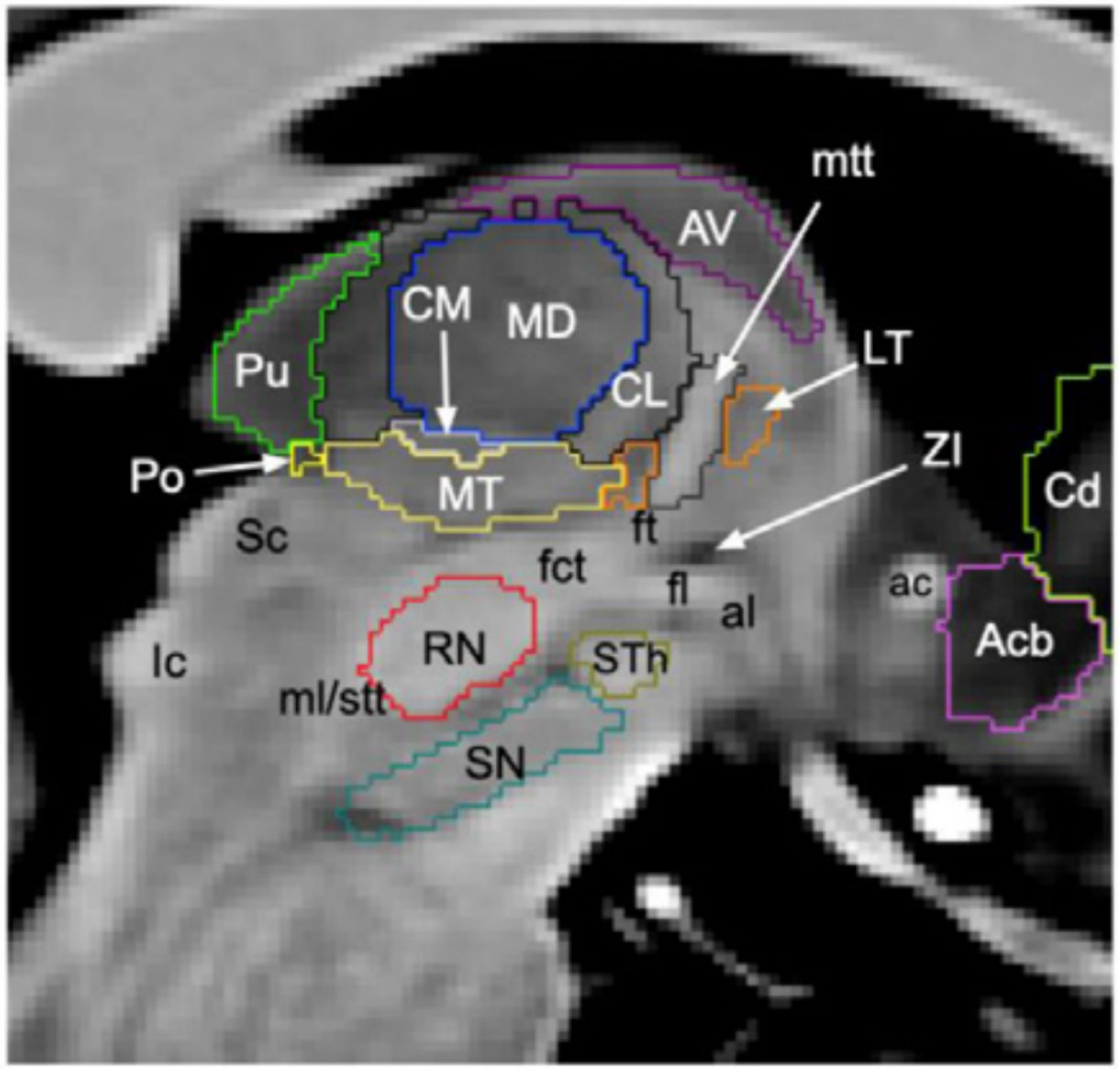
Segmentation of thalamic nuclei. Sagittal slice of T1-weighted 7 T brain magnetic resonance imaging where the thalamic nuclei most frequently implanted in SEEG are shown, i.e., pulvinar (Pu, green), centromedian (CM, grey), mediodorsal (MD, blue) and anterior nuclei (AV, purple). Figure from Brun et al. (78).
